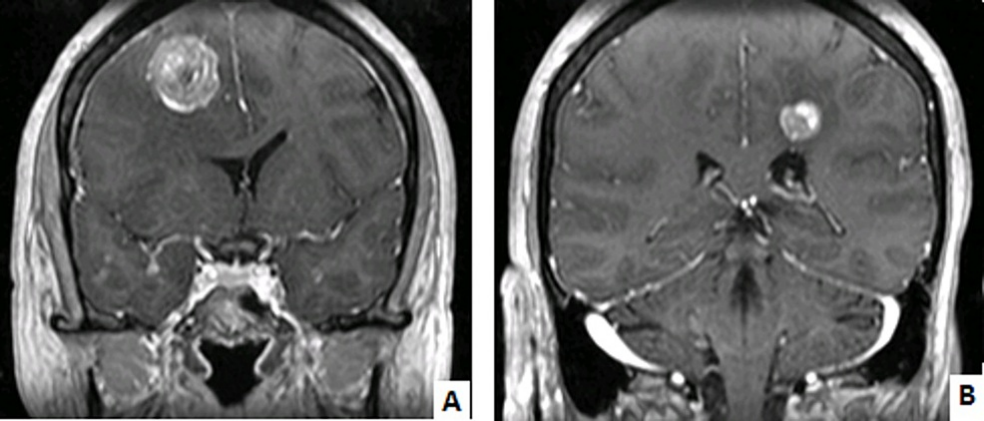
Cranial Magnetic Resonance imaging at brain recurrence A: Right frontal lobe lesion with 29 x 24 mm, oedema, and midline deviation. B: Left front parietal lobe lesion with 16 x 14 mm.
